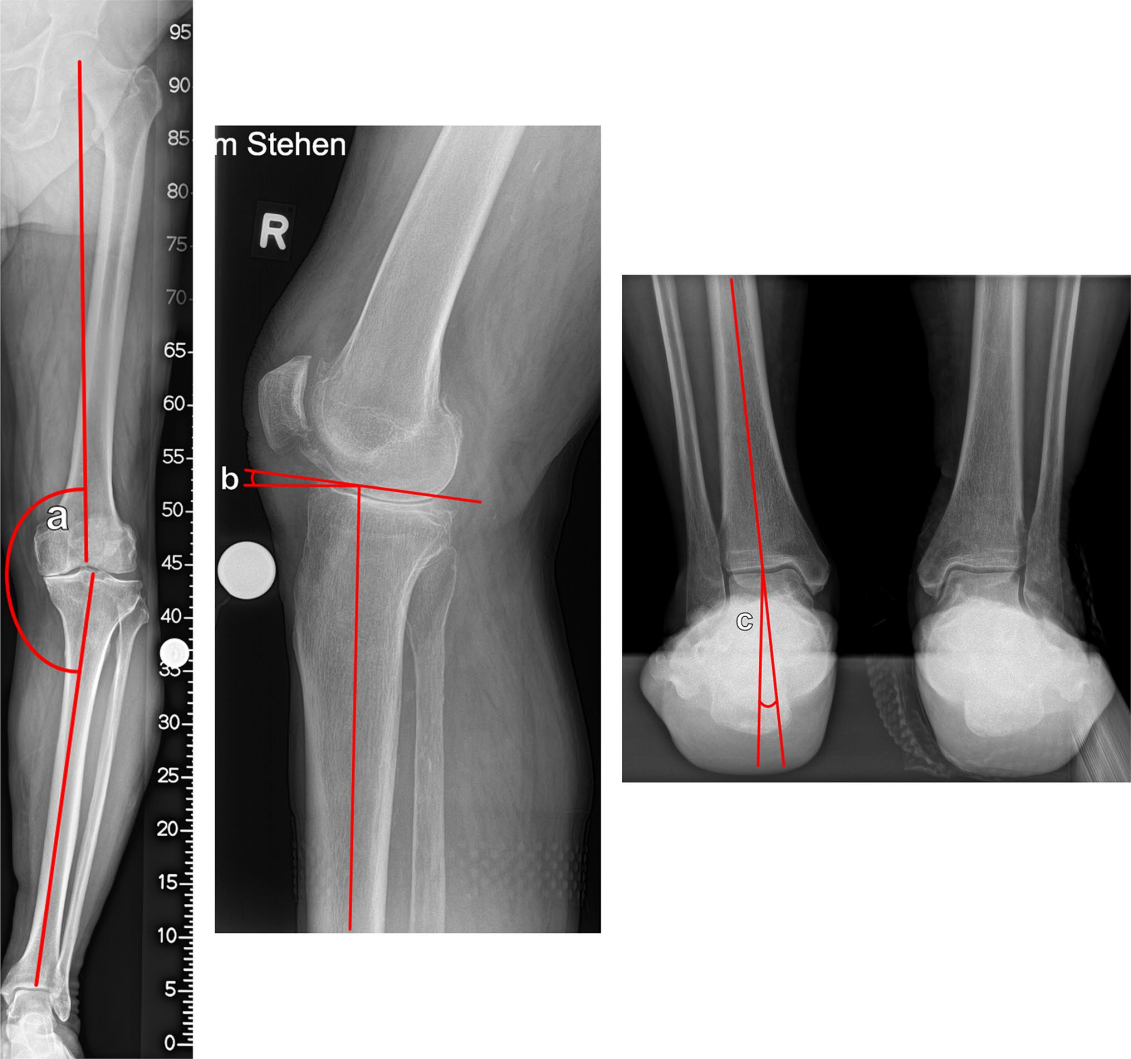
a The mTFA (a) was defined as the angle between the mechanical femoral and mechanical tibial axes. The mLDFA (b) was the angle between a tangent to the subchondral distal femoral condyles and the mechanical femoral axis. The MPTA (c) was the angle between the tangent to the proximal tibial joint surface and the mechanical tibial axis. b The slope (d) was measured as suggested by Dejour et al. [4, 11]. c The HAVA (e) was the intersection between the mechanical tibial axis and a line connecting the most distal point of the calcaneus and the centre of the ankle joint in a standing hindfoot radiograph
